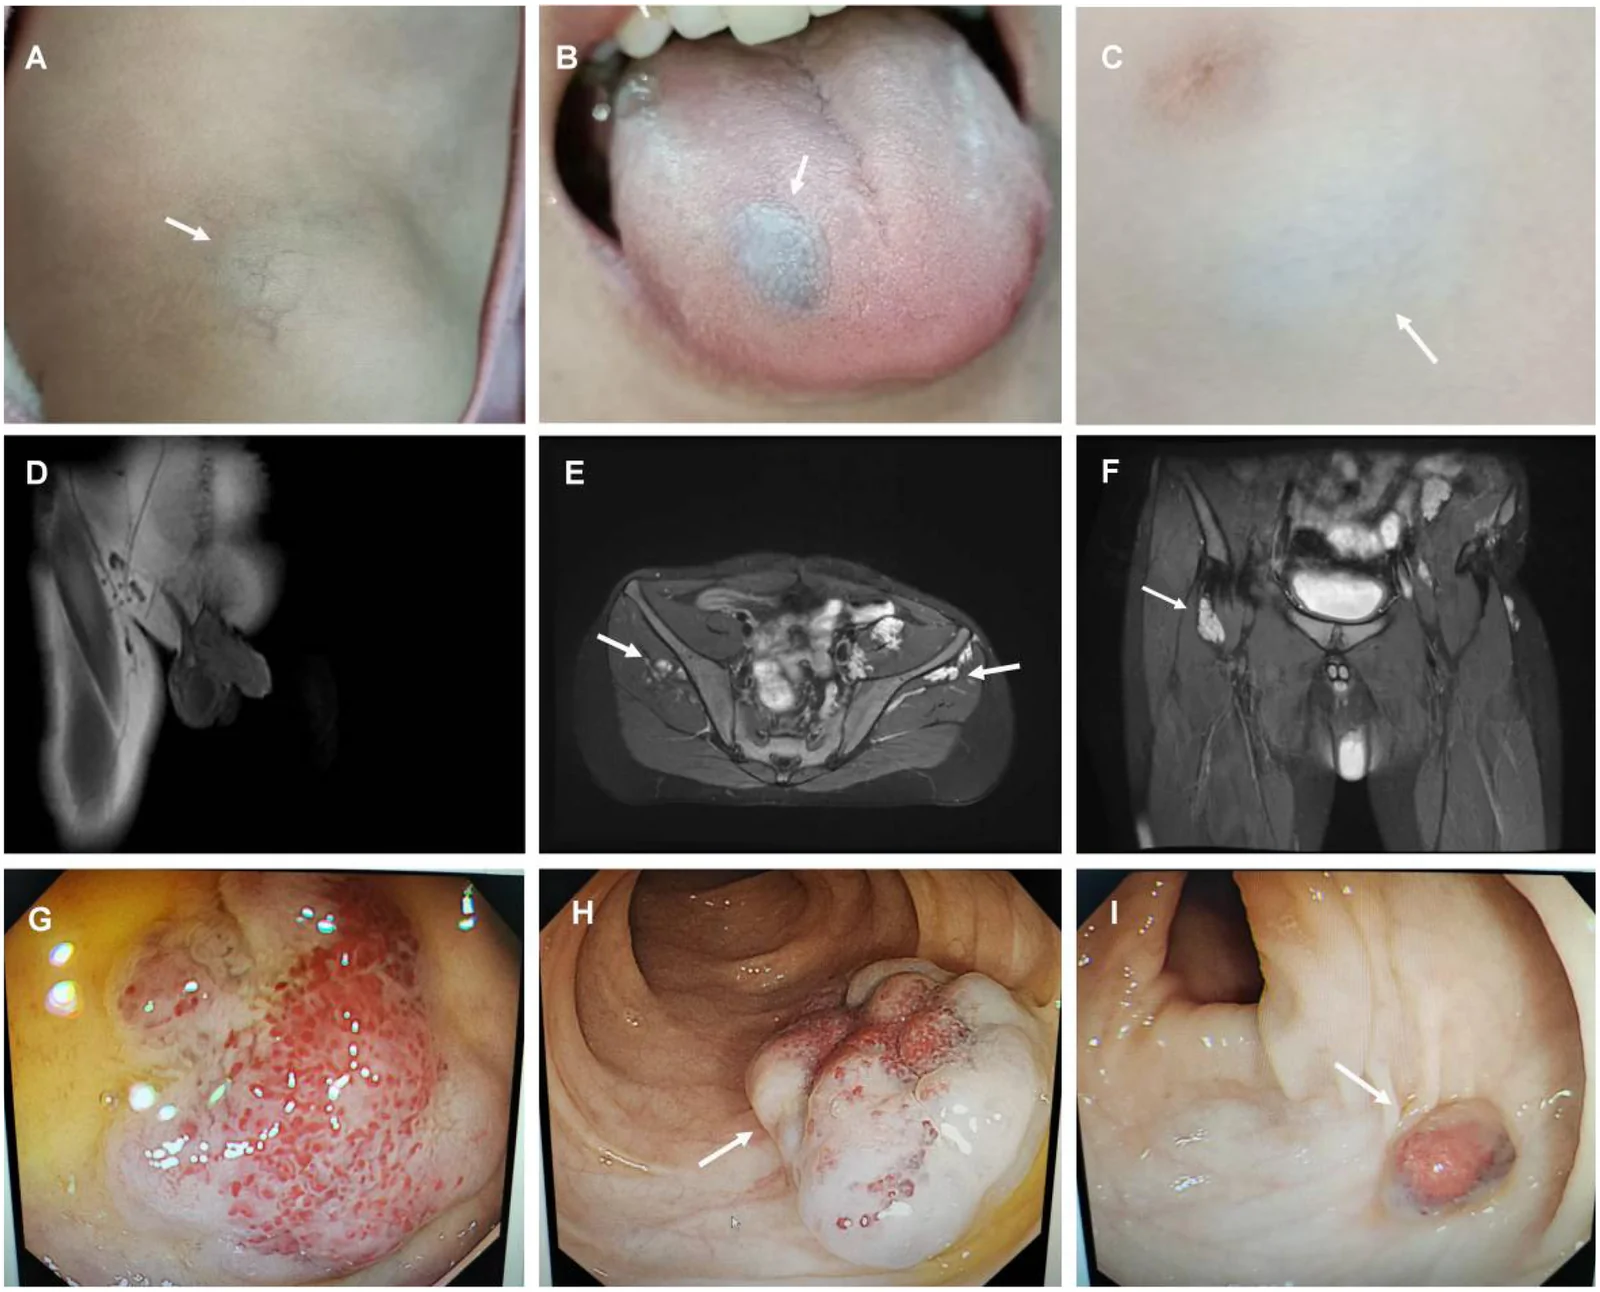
Clinical, magnetic resonance imaging, and endoscopic manifestations of multifocal venous malformations in blue rubber bleb nevus syndrome. (A–C): Clinical photographs showing representative bluish superficial venous malformations involving the skin and tongue. (D): Magnetic resonance imaging showing a large lobulated superficial soft-tissue venous malformation in the gluteal/proximal thigh region. (E): Axial magnetic resonance image showing multiple venous malformations involving the pelvic and gluteal soft tissues. (F): Coronal magnetic resonance image showing multifocal venous malformations involving the pelvic and proximal thigh soft tissues bilaterally. (G): Gastroscopy showing a 0.3-cm mucosal venous malformation with superficial ectatic vessels along the greater curvature of the gastric body. (H): Colonoscopy showing a protruding reddish-blue venous malformation in the ascending colon. (I): Colonoscopy showing a similar venous malformation in the rectum. White arrows indicate representative lesions in panels A-C, E, F, H, and I. The endoscopic and magnetic resonance images in panels D-I were obtained during the hospitalization at 17 years of age.
